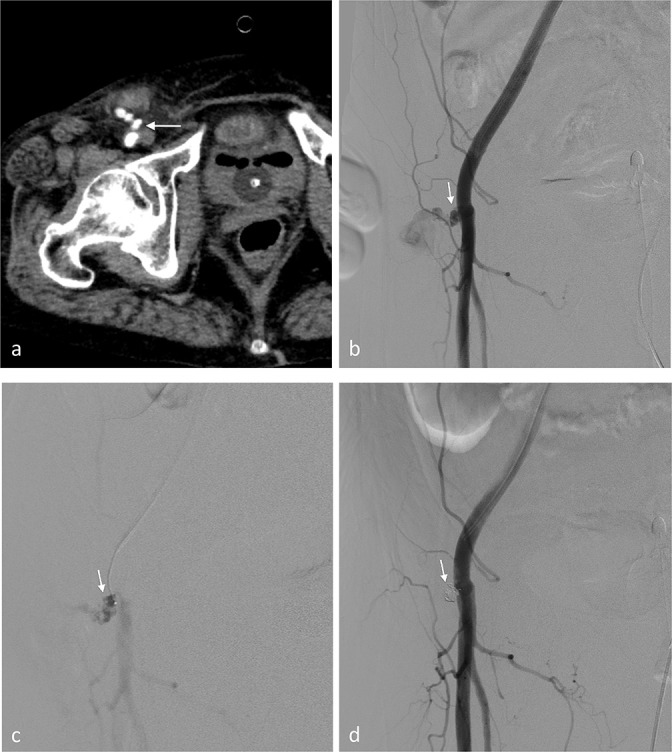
(Case 1) Common femoral pseudoaneurysm after mechanical thrombectomy for a superior mesenteric embolism. (a) CE-CT reveals a progressive pseudoaneurysm (arrow) of the right femoral artery. (b) Right femoral arteriogram via the contralateral femoral approach. The left anterior oblique (LAO) 50 projection shows right common femoral pseudoaneurysm (arrow). (c) The pseudoaneurysm (arrow) is cannulated using a 0.019-inch microcatheter with an angle-shaped tip (Progreatλ; Terumo Corporation, Tokyo, Japan). (d) After the deployment of detachable coils (2–2.5 mm diameter; Target, Stryker Neurovascular, CA, USA) into the aneurysmal neck, the final angiogram shows the exclusion of the pseudoaneurysm (arrow).
